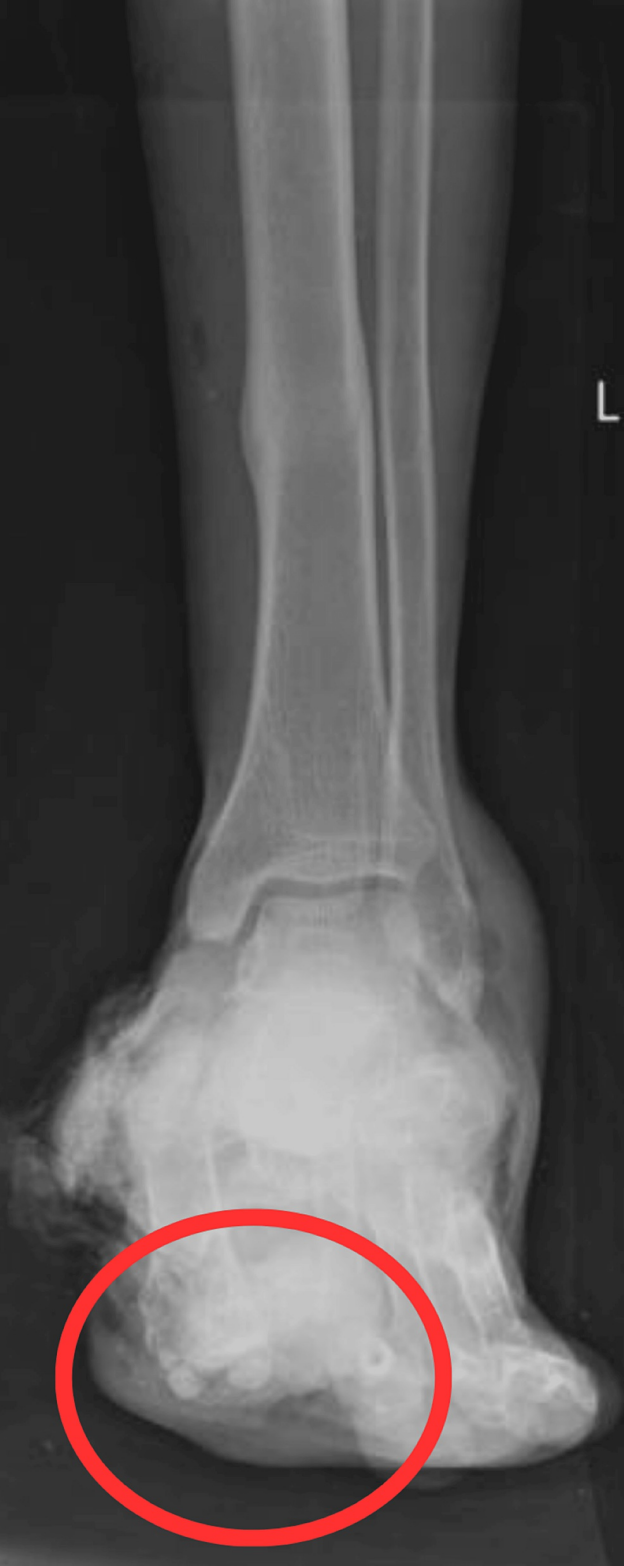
PA view of X-ray of the affected foot The circle shows the heel pad avulsion PA, posteroanterior
